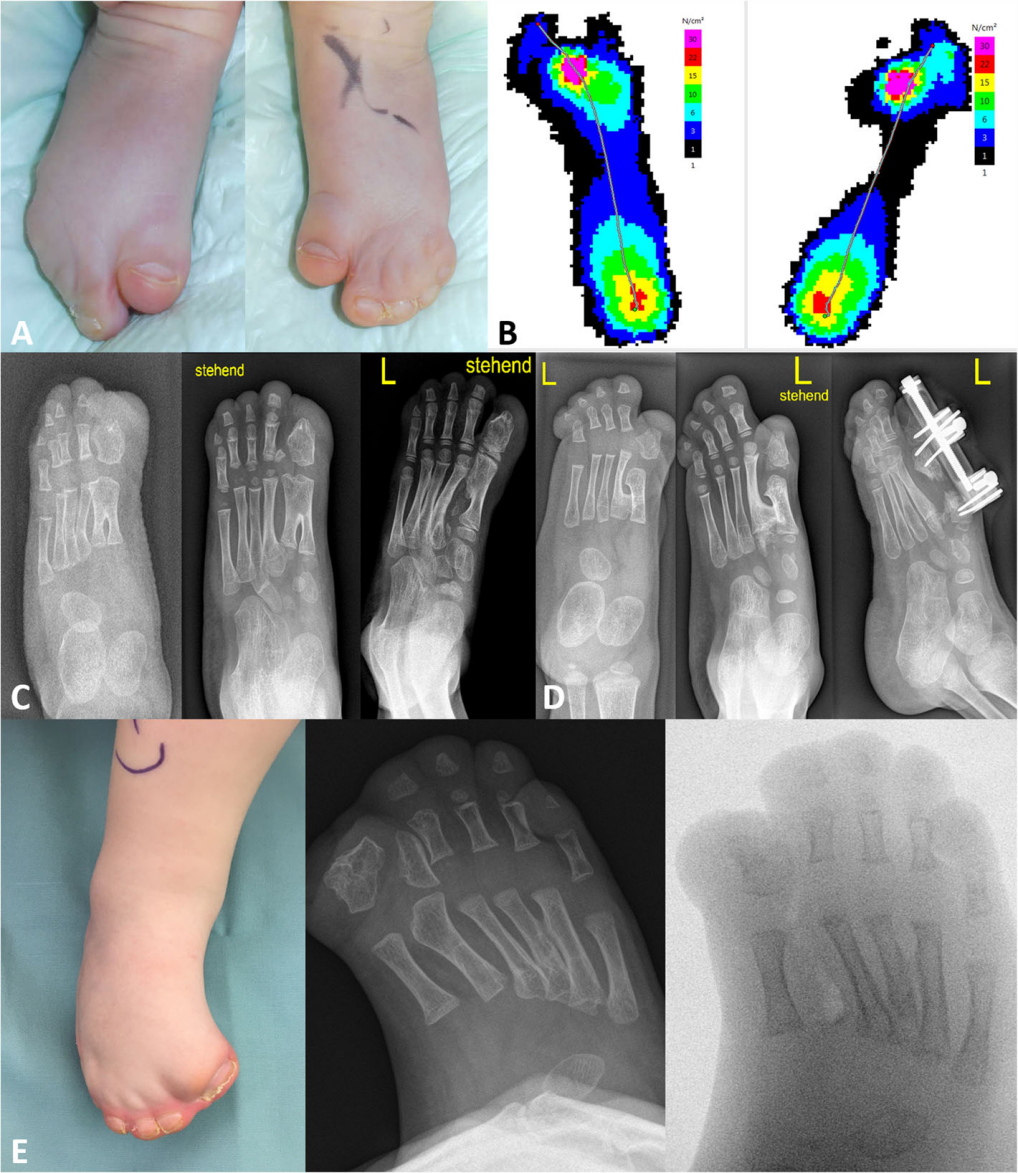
Left and right foot of a 3-year old boy with Apert’s syndrome showing complex syndactyly of toes 2 to 4, as well as a shortened and medially deviating great toe as a typical deformity (a). Pedobarography of a 4-year-old boy with Apert’s syndrome. Pressure distribution shows a shift laterally, with the maximum weight bearing along the metatarsals 3 and 4 on the left foot (84,7 N/cm^3^). The right foot shows a similar shift of the pressure points, the maximum is on the third metatarsal with a smaller weight bearing area (95 N/cm^3^) (b). Images of a 6-month old child with an interposed additional ray are shown. At the age of 4 years one can depict the calcaneocuboidal fusion, a synchondrosis between the metatarsal III base and the distal tarsals as well as an ossification between the great toe and the second toe. The final image after surgery 2 years postoperatively is shown. The additional full ray was resected, and a metatarsal II elevation osteotomy was performed to reduce the plantar pressure below it (c). Images of another case, 1 year and then 5 years of age, reveals an osseous bridge between metatarsal I and II, marked shortening of the first ray and mild hallux varus. A gradual lengthening procedure was performed to elongate the shortened ray (d). Images of a case (Blauth type III) with complete syndactylies I-V and forefoot adductus are shown. The radiographs show marked forefoot adduction partially caused by an interposed supernumerary dysplastic ray. Following resection of this ray the forefoot position and shape was markedly improved (e)
